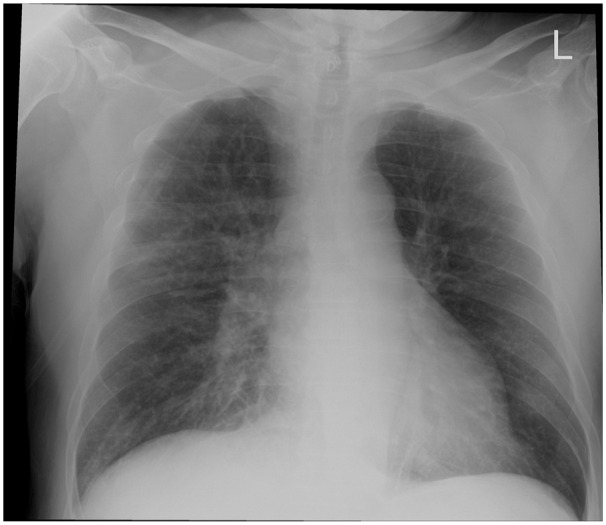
Chest X-ray showing hazy opacification of hila and central vascular congestion with prominent hazy interstitial markings suggestive of pulmonary edema.
